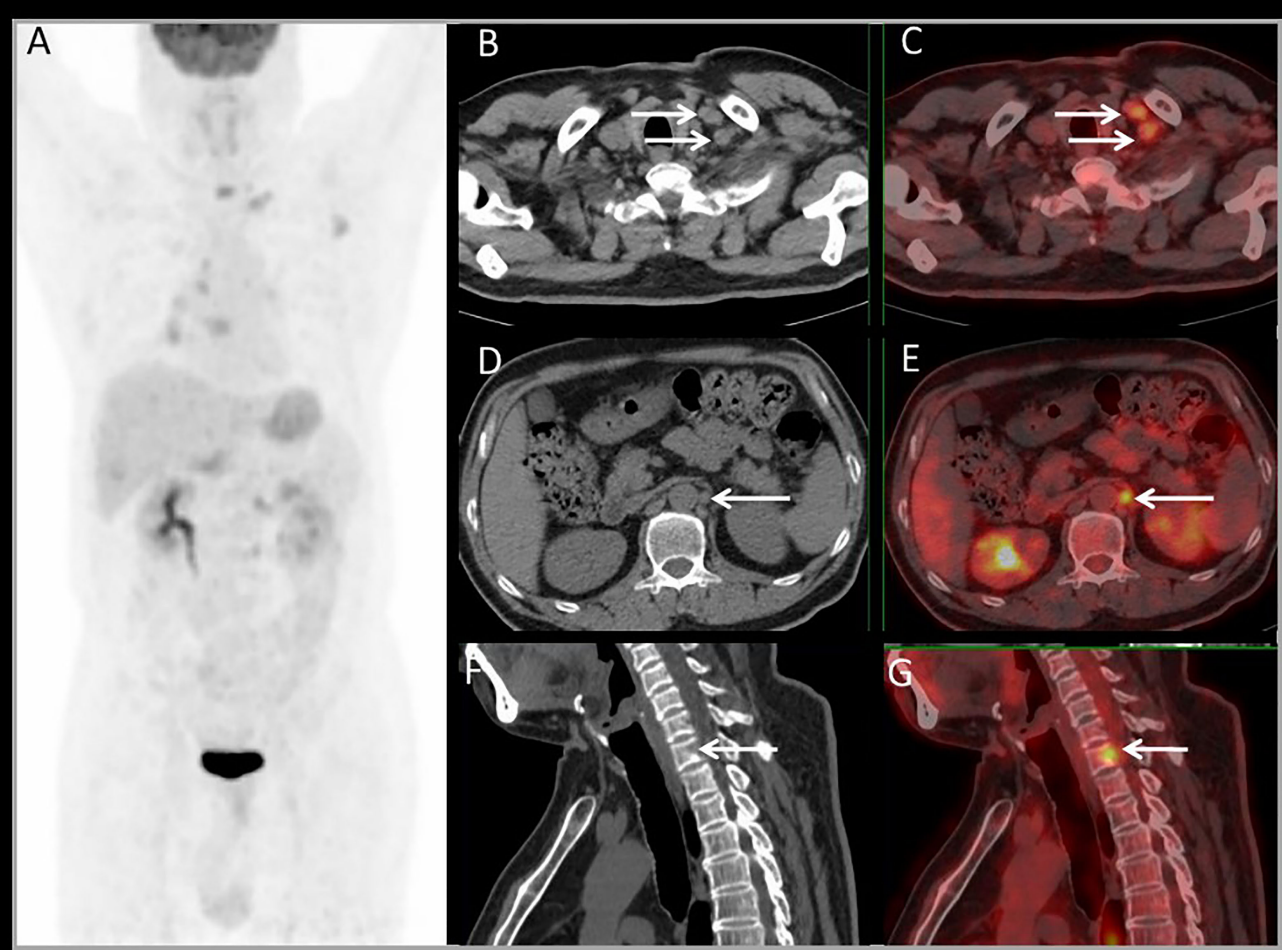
Images of a 54-year-old male with rectal liver metastases. Common CT showed no EHD. The MIP (A) of PET/CT showed liver metastasis and multiple EHD. PET and PET/CT fusion images showed left supraclavicular lymph nodes metastases (B, C), retroperitoneal lymph nodes metastases (D, E), and bone metastases on the 7th cervical vertebra (F, G). The therapy strategy was changed from resectable to unresectable (major impact). The patient received systematic therapy after PET/CT restaging.
